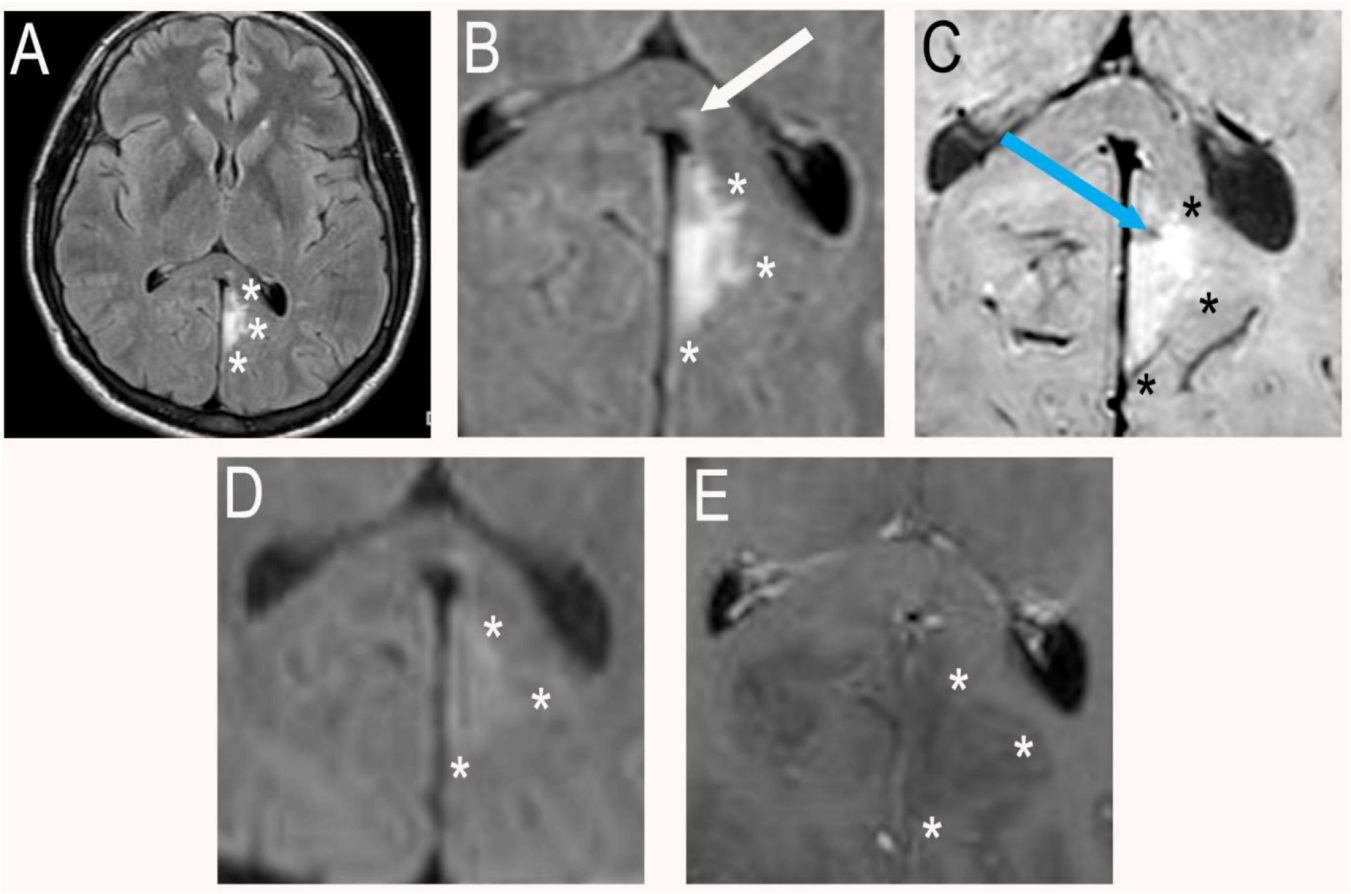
Axial T2 FLAIR (A) showing hyperintense triangular area within medial left occipital lobe and abutting the falx (white asterisks) corresponding to lesion on the prior head CT. There is also a punctate area of hyperintensity within the splenium of the corpus callosum on the cropped axial T2 FLAIR image (B) (white arrow). Cropped axial SWI (C) showing faint hyperintensity in the medial left occipital lobe (black asterisks) and punctate hypointensity anteriorly which may represent focal hemorrhage (light blue arrow). There was no diffusion restriction at the time of scanning (D) with hyperintensity corresponding to shine through (white asterisks) and Finally, axial T1 post contrast enhanced image (E) showing no enhancement of the lesion (white asterisks).
